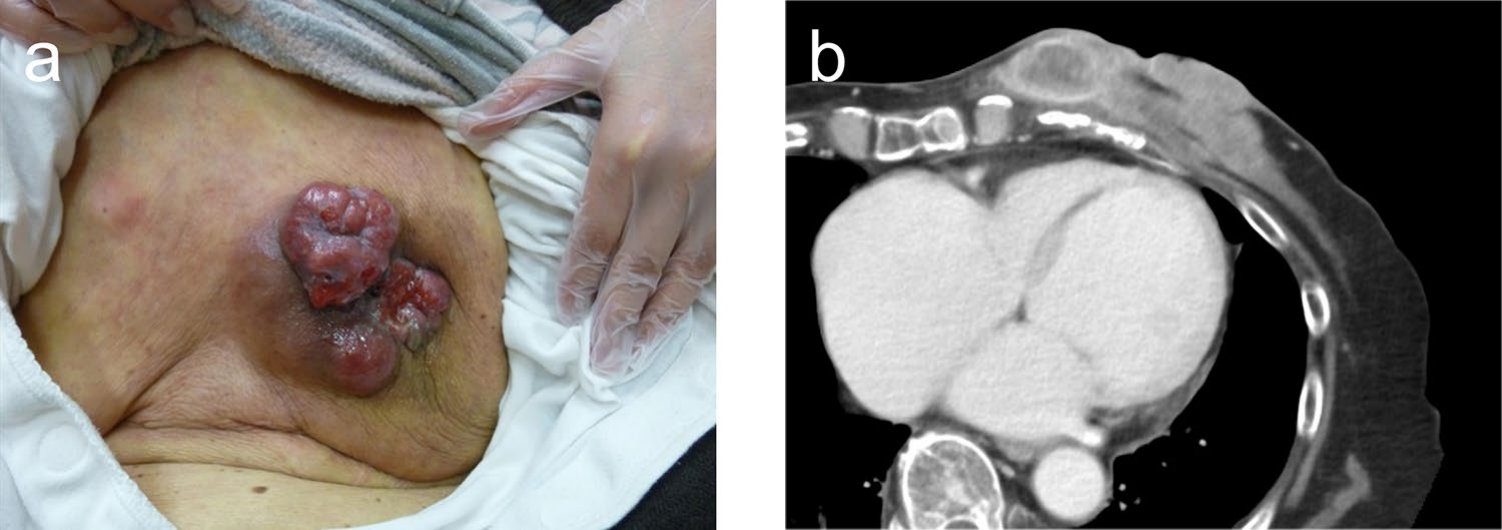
a A physical examination and b a CT scan have revealed the recurrence of the breast mass, which was observed 11 months following the completion of radiation therapy
